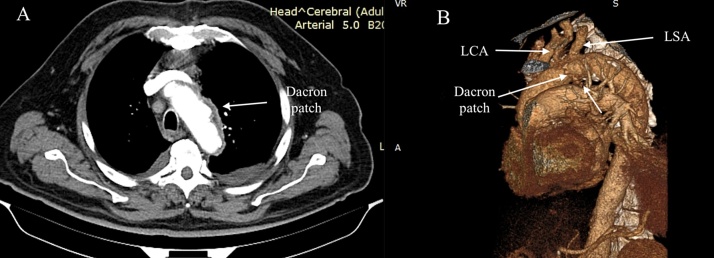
Multislice 3D reconstruction CT scan of the aortic arch after three months showing no evidence of filling or enlargement of the AAP. (AAP- aortic arch pseudoaneurysm).
